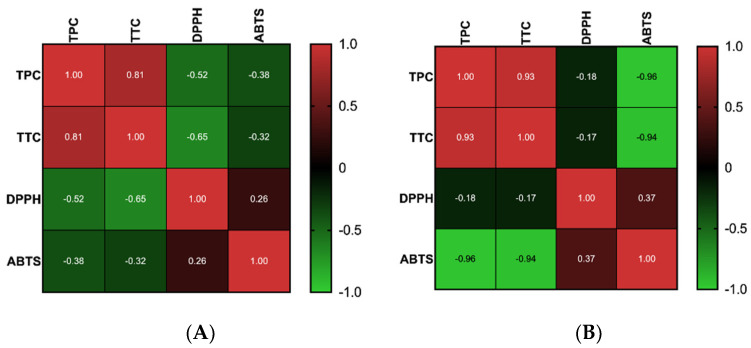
Pearson’s correlation coefficients among TPC, TTC, DPPH, and ABTS calculated for the extracts obtained by microwave-assisted extraction (A) and by ultrasound-assisted extraction (B).
